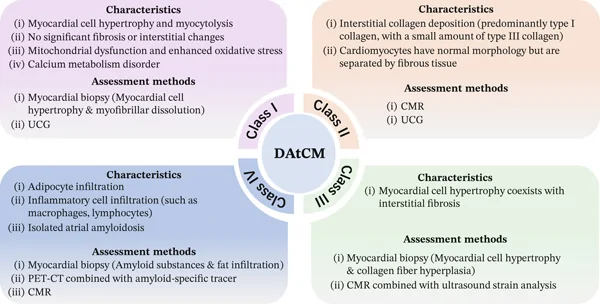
Characteristics and assessment methods for each EHRAS class. UCG, ultrasonic cardiogram; CMR, cardiac magnetic resonance; PET‐CT, positron emission tomography–computed tomography.
